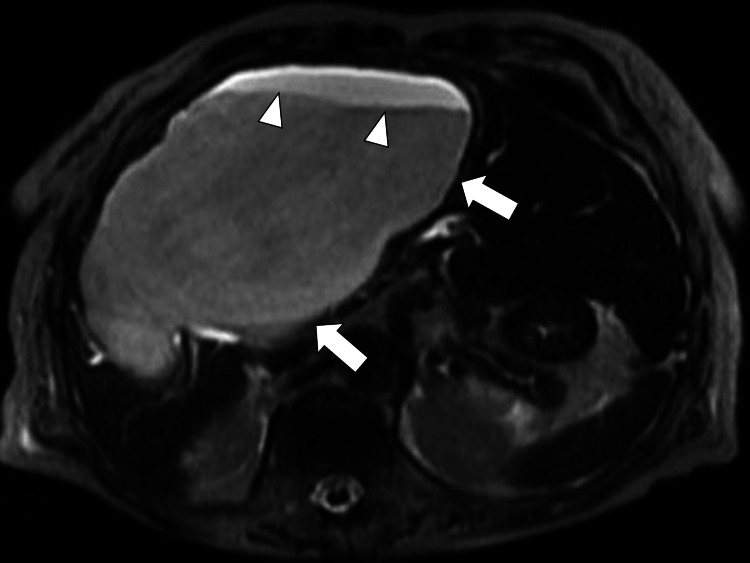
T2-weighted magnetic resonance imaging showing the hepatic cyst (white arrows) and debris (white arrowheads)
